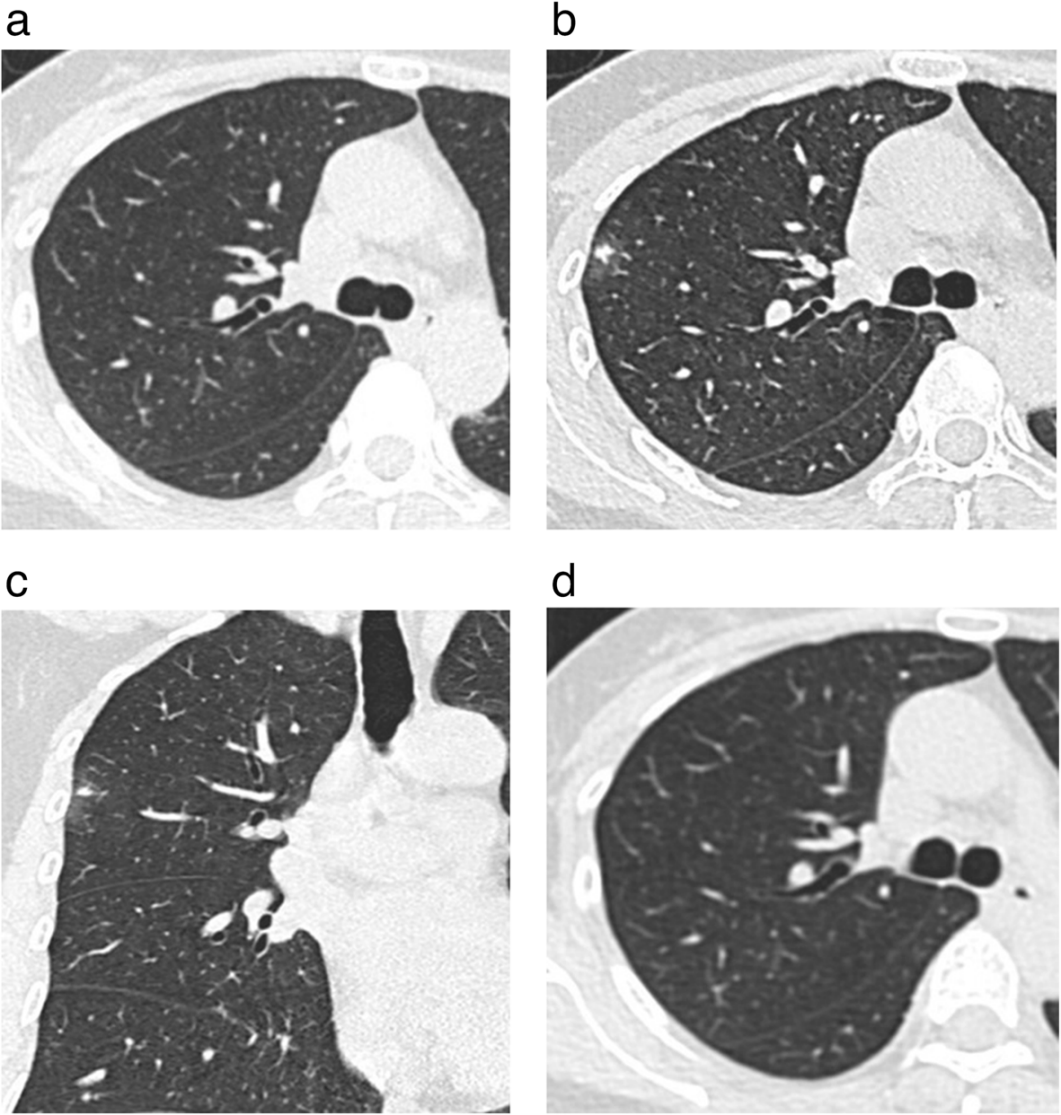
Absorbable benign pulmonary subsolid nodule in a 48-year-old woman. a The initial axial lung window CT image showed a normal right upper lobe. b, c Four months later, the axial and coronal lung window CT images showed a subsolid nodule with an ill-defined margin and a target sign in the right upper lobe. d Ten months later, the axial lung window CT image showed that the nodule was resolved
